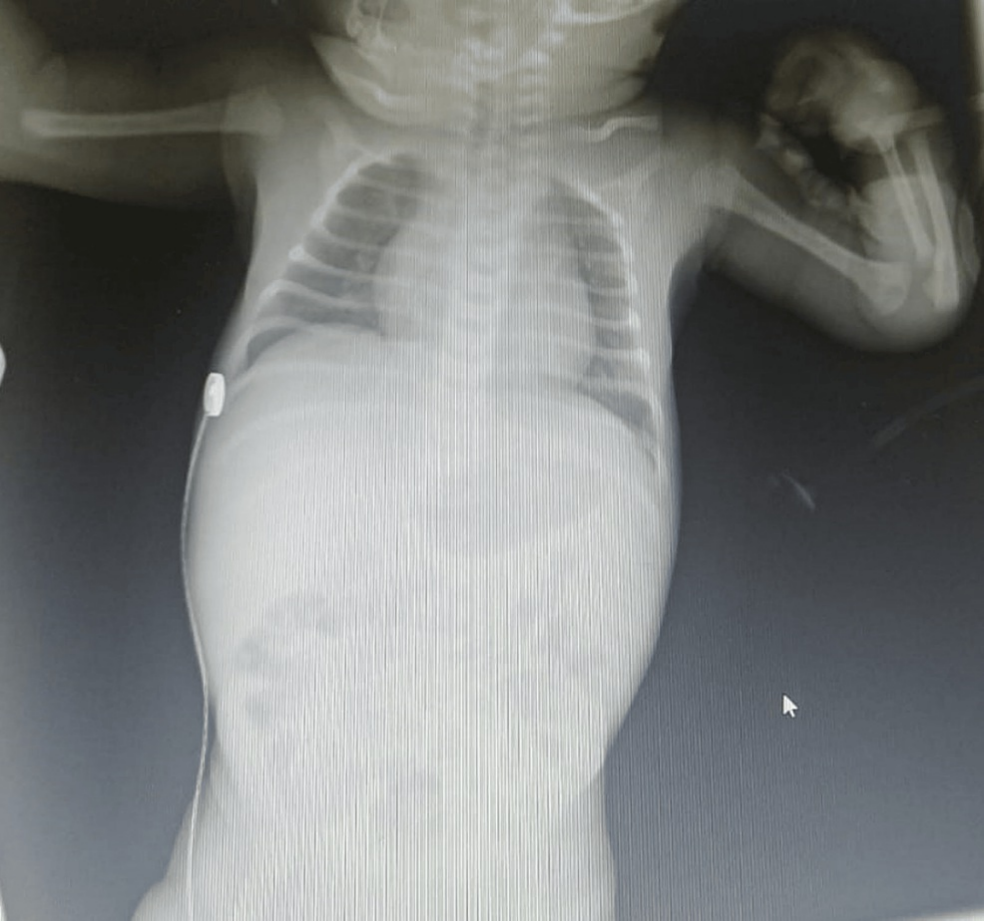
X-ray image of the patient's chest
